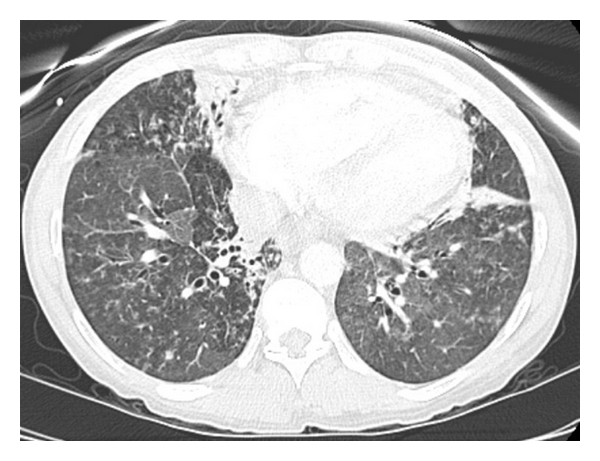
Computed tomography of the thorax showing extensive nodular, reticular, and groundglass opacities in both lungs along with mediastinal enlargement.
